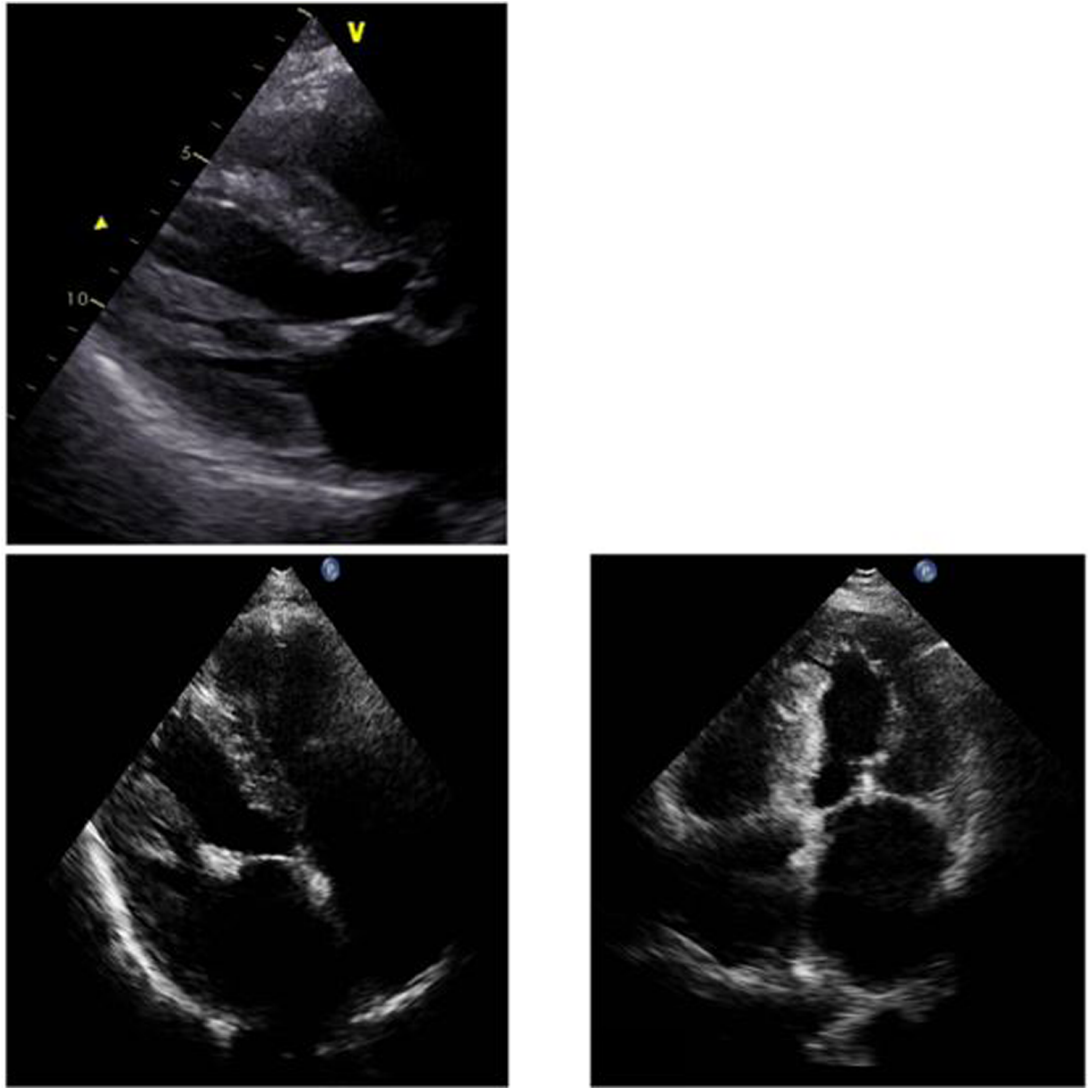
Myocardial thickening and dilated atria in the parasternal long axis and in the apical four chamber view.
